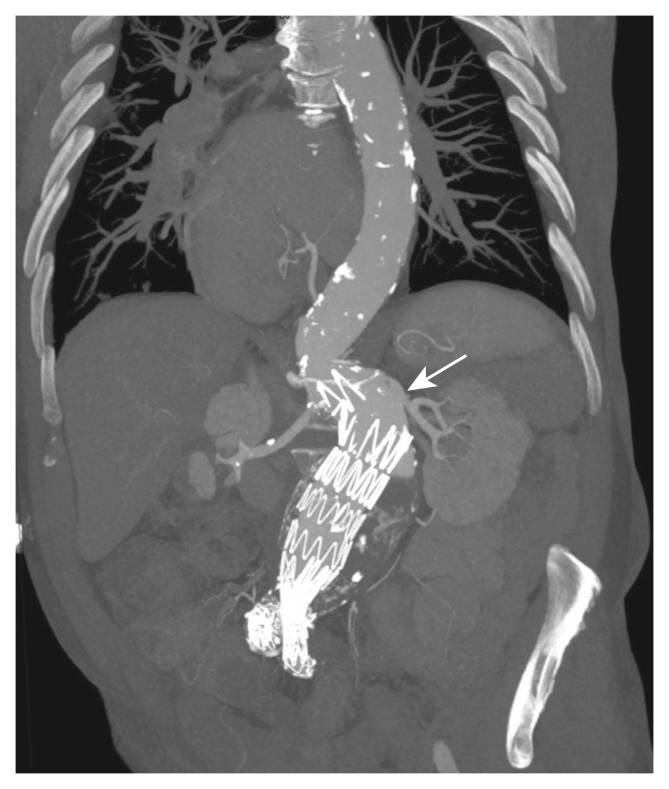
Computed tomography angiogram (coronal plane) of a 74 year old male patient with abdominal aortic aneurysm showing endovascular graft suprarenal bare metal stent separation (white arrow).
